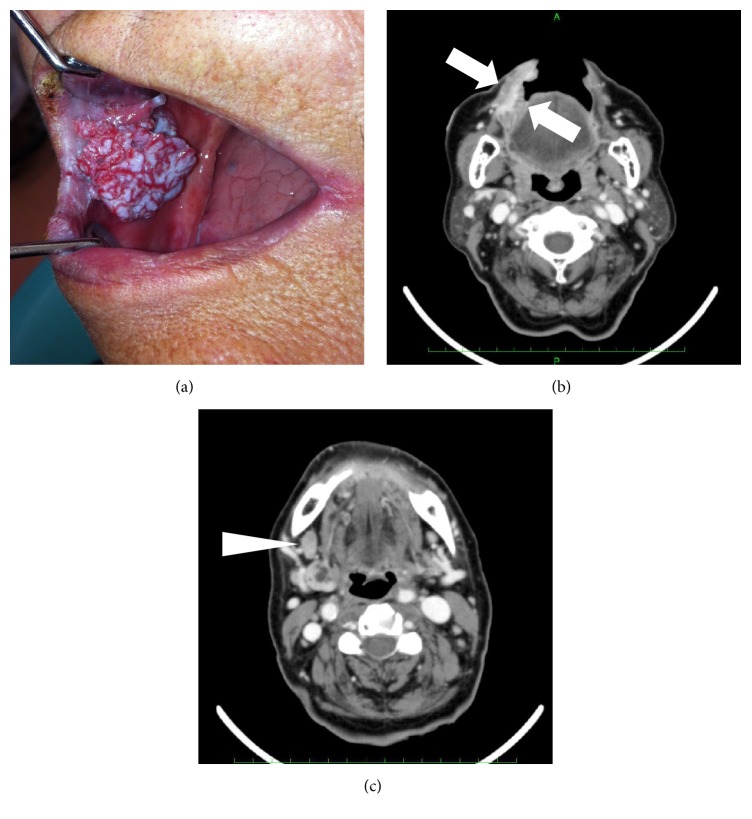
Intraoral findings at initial presentation*. Ulcerated lesion in the right buccal area (a). CT images in the right buccal area show an enhanced lesion ((b), arrow) and enlarged submandibular lymph node ((c), arrow head).
